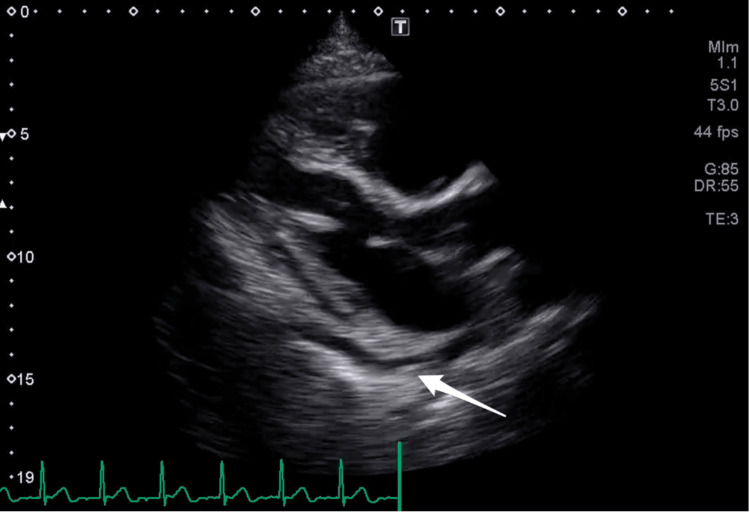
Small pericardial effusion (white arrow) visualized in the parasternal long-axis view of TTE TTE: transthoracic echocardiogram
